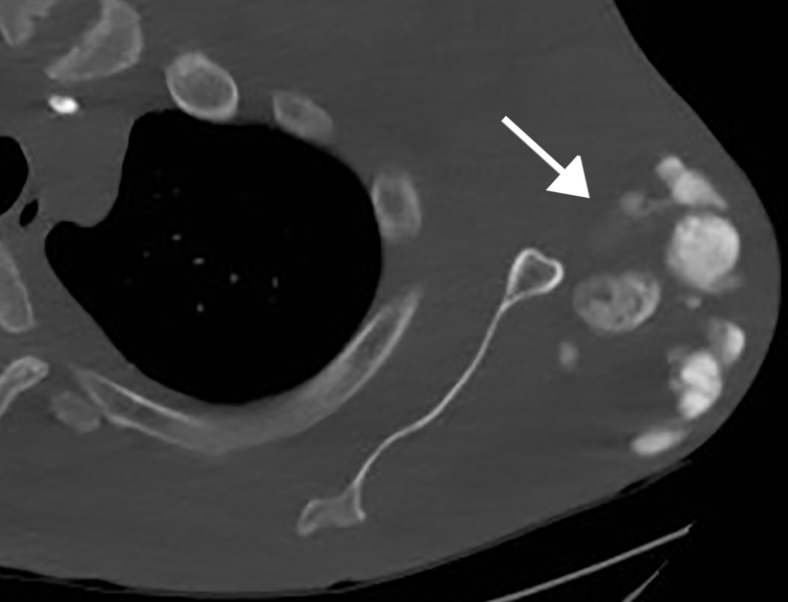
Left shoulder CT Axial CT image at the level of the left shoulder demonstrates dense multilobulated periarticular masses (arrow). Note lack of osseus destruction in the adjacent left scapula.
